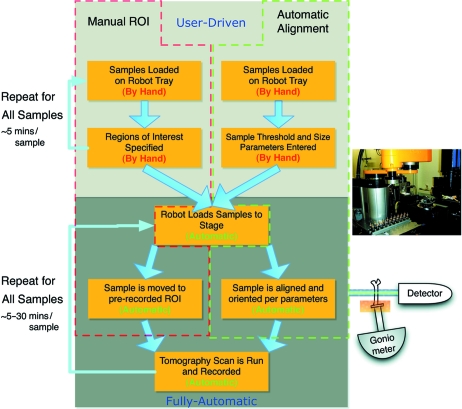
The flow of an experiment using the automated systems at TOMCAT. The two modes of operation Manual ROI (red) and Automatic Alignment (green) are shown. The Manual ROI mode is for samples where the measurement regions must be manually defined by the user, and the sample exchange and scanning is run automatically. The Automatic Alignment mode, once the thresholds have been set, is completely automatic, requiring only that the user mounts the samples on the tray and clicks start. The system will for every sample load with the robot, align using the X-ray beam and scripts, and scan.
